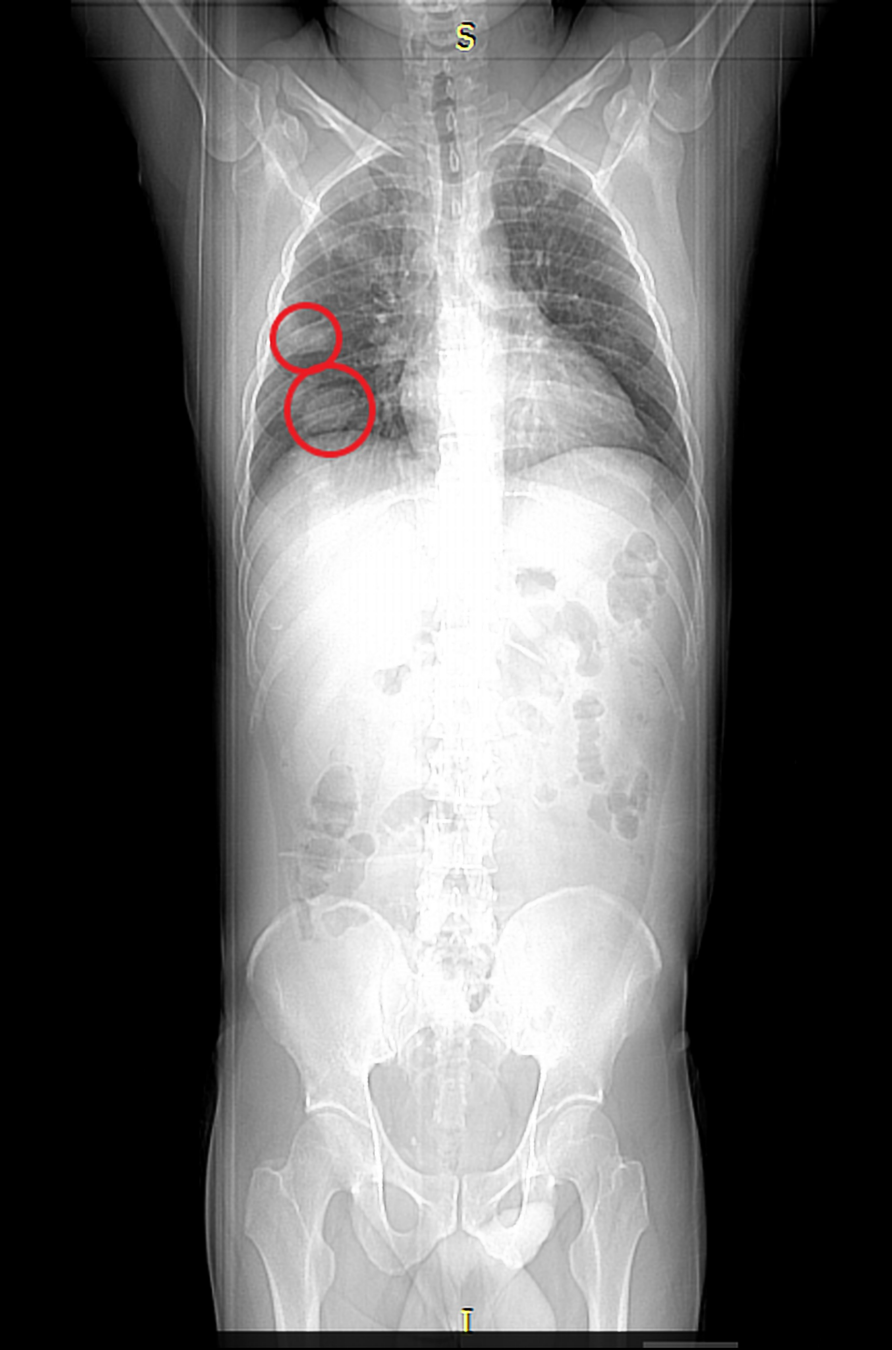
Scan projection radiograph of the anterior chest showing pulmonary nodules (red circles)
